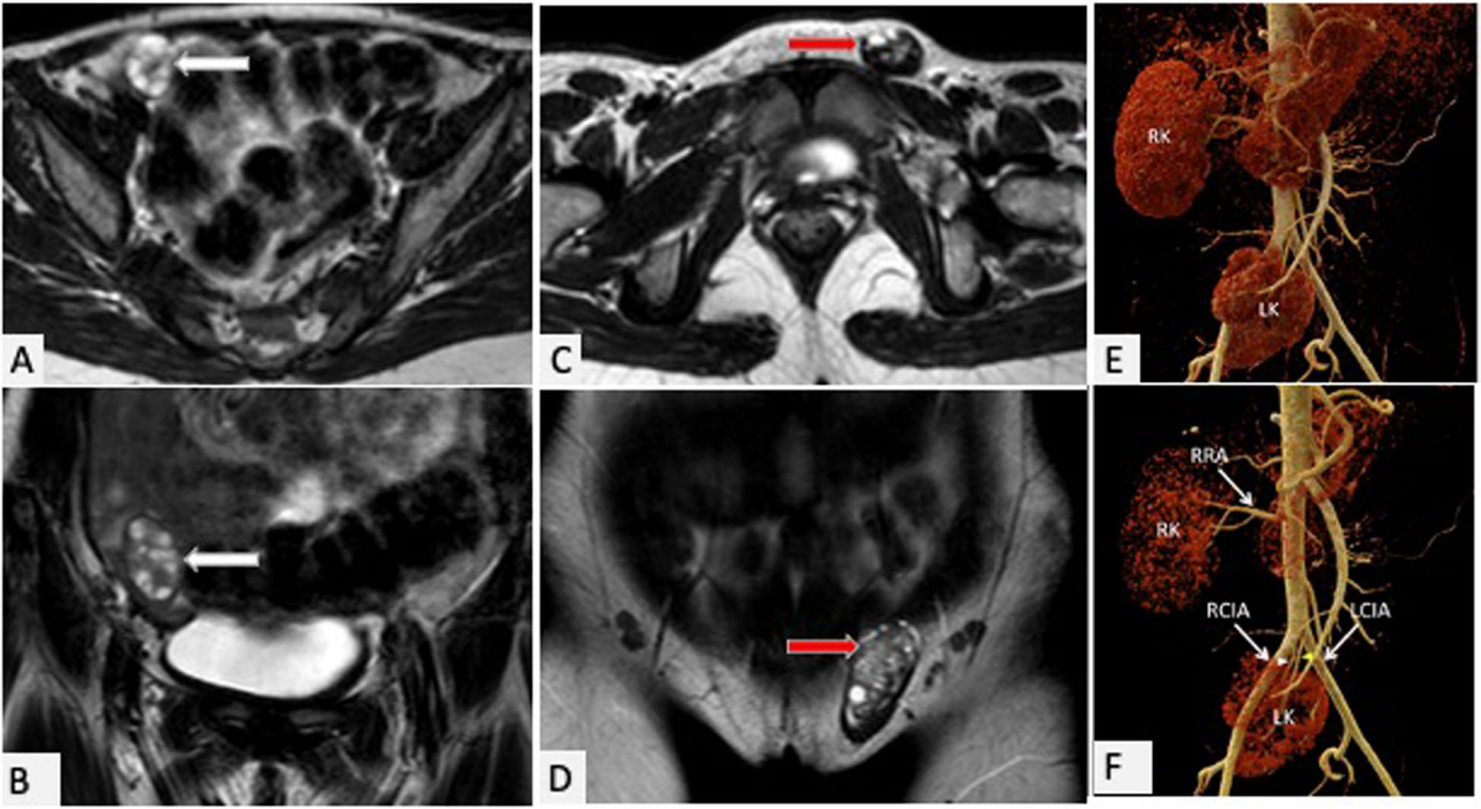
MRI images (A, B) showing axial and coronal T2w images showing anteriorly located right ovary (white arrows) at the internal inguinal ring. MRI images (C, D) showing axial and coronal T2w images showing left ovary (red arrows) located in the left inguinal canal. Volume rendered images of computed tomography angiography (E and F) show the left kidney (LK) in the pelvis with two branches (indicated by white and yellow arrowheads respectively) arising from the right common iliac artery (RCIA) and the left common iliac artery (LCIA) respectively seen supplying the ectopic left kidney. The right kidney (RK) was normally located with a normal right renal artery (RRA)
